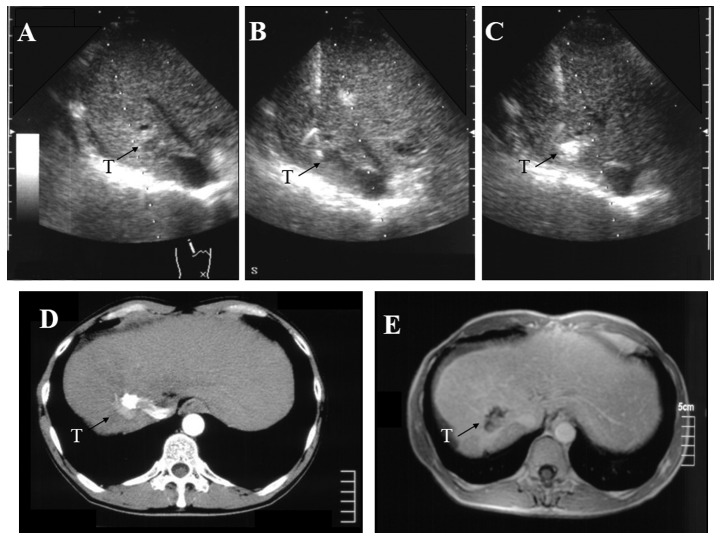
Image findings for patient 2. (A) Ultrasonograms captured by right intercostal scanning prior to therapy. A right portal vein (PV) intersects the puncture point and a low-echoic nodule prior to therapy (arrow). (B) A needle tract of an overcoat needle and an extended curved percutaneous ethanol injection therapy (PEIT) needle from the overcoat needle are observed at the right side of the right PV. A curved PEIT needle deviates around the vessel to the cancer nodule and is inserted in the right side of the tumor (arrow). (C) Immediately following the ethanol injection, the tumor area undergoes a hyperechoic change (arrow). (D) A small enhanced nodule (1.3×1.3 cm) is located in S7 in the arterial phase of an abdominal dynamic computed tomography image prior to therapy I (arrow). (E) Arterial phase image of the abdominal dynamic magnetic resonance imaging scan that was obtained subsequent to the therapy using a curved PEIT needle. The treated area evolved into a low-intensity area following therapy (arrow).
